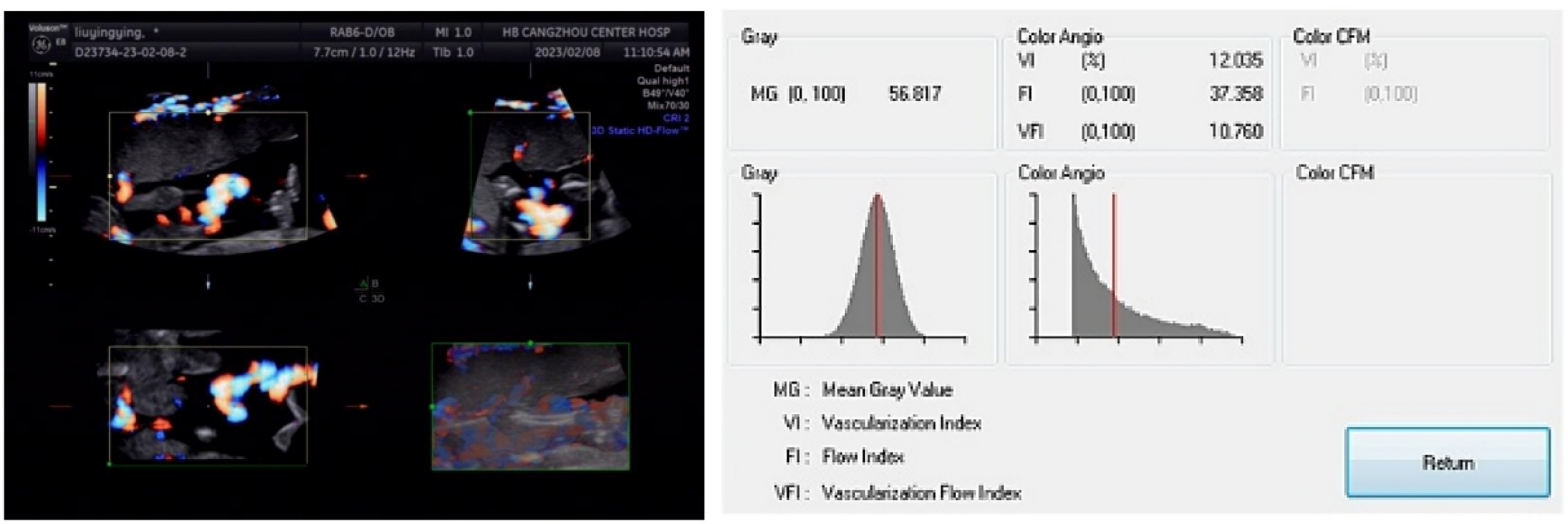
Representative spherical contour of the placenta measured by three-dimensional power Doppler ultrasonography and the output of the vocal analysis. CFM: Color flow mode.
